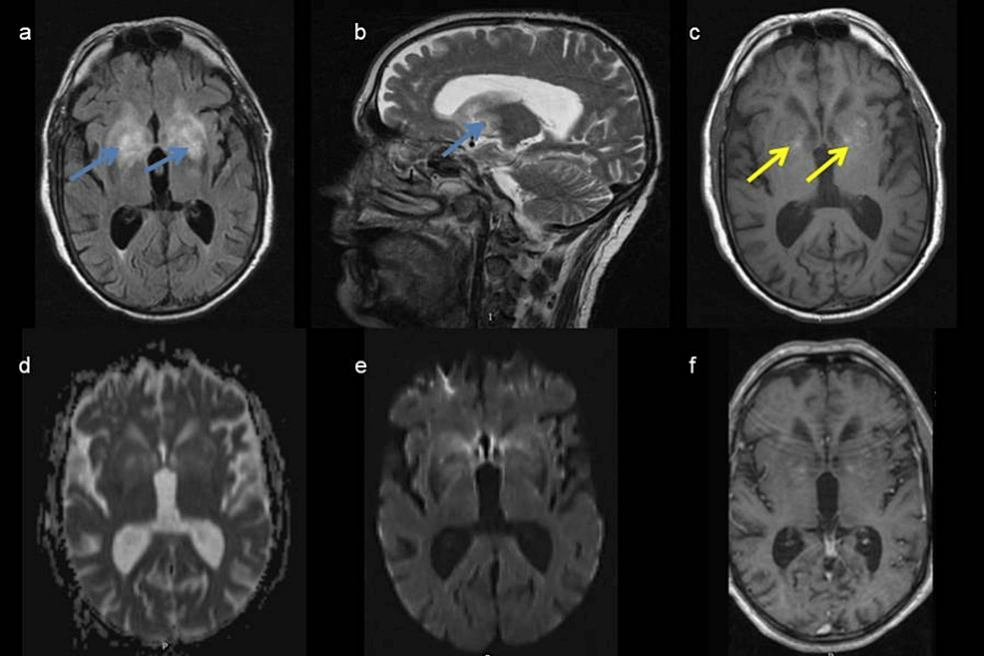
Brain magnetic resonance imaging in the acute phase showing bilateral lenticulocapsular and caudate nuclei hyperintensity (blue arrow) on T2/fluid-attenuated inversion recovery (a, b), hyperintensity foci on T1 sequence (c, yellow arrow) without restriction to diffusion (d) or contrast enhancement (e, f).
